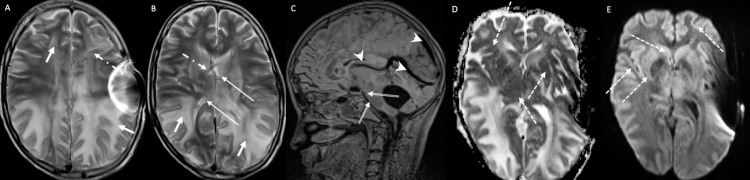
MRI of the brain. (a, b) Bilateral parieto-occipital, temporal, and bifrontal high T2 white matter signal changes (short arrows) in keeping with posterior reversible encephalopathy syndrome (PRES). The tip of the left frontal approach EVD (dash-dot arrows) is seen within the right lateral ventricle associated with the collapsed ventricular system (long-arrow) in keeping with over shunting. (c) Sagittal FLAIR demonstrates engorged dural venous sinuses and deep cerebral veins (arrowheads), flatted belly of the pons, and reduced mammilopontine angle = 1 mm (double arrows), these findings represent sequelae of intracranial hypotension secondary to over shunting. (d, e) Diffuse cerebral cortical and deep ganglionic restricted diffusion (dashed arrows) suggestive of hypoxic-ischemic encephalopathy. EVD - extra-ventricular shunt drain
